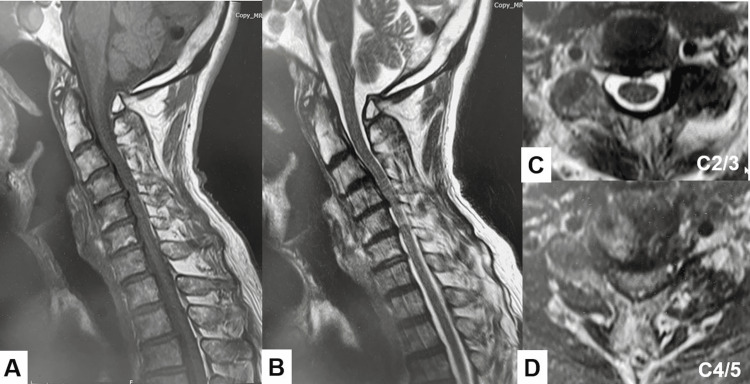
Preoperative MRI. A. T1-weighted mid-sagittal image. B. T2-weighted mid-sagittal image. C. T2-weighted axial image at C2/3. D. T2-weighted axial image at C2/3. The spinal cord was severely compressed at the C4/5 level.
